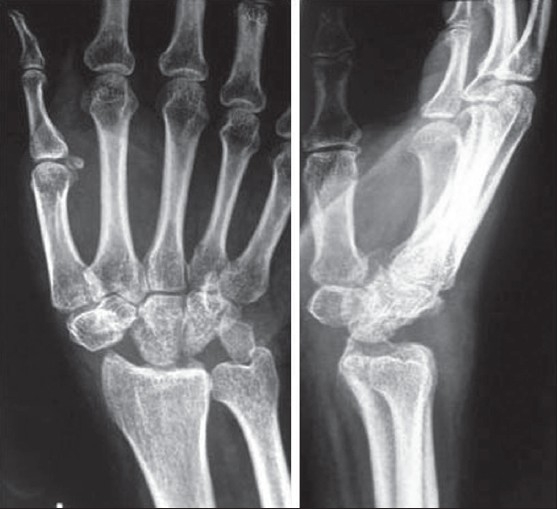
Anteroposterior and lateral radiograph of left wrist at 12 months follow-up shows alignment of carpus on distal radius
